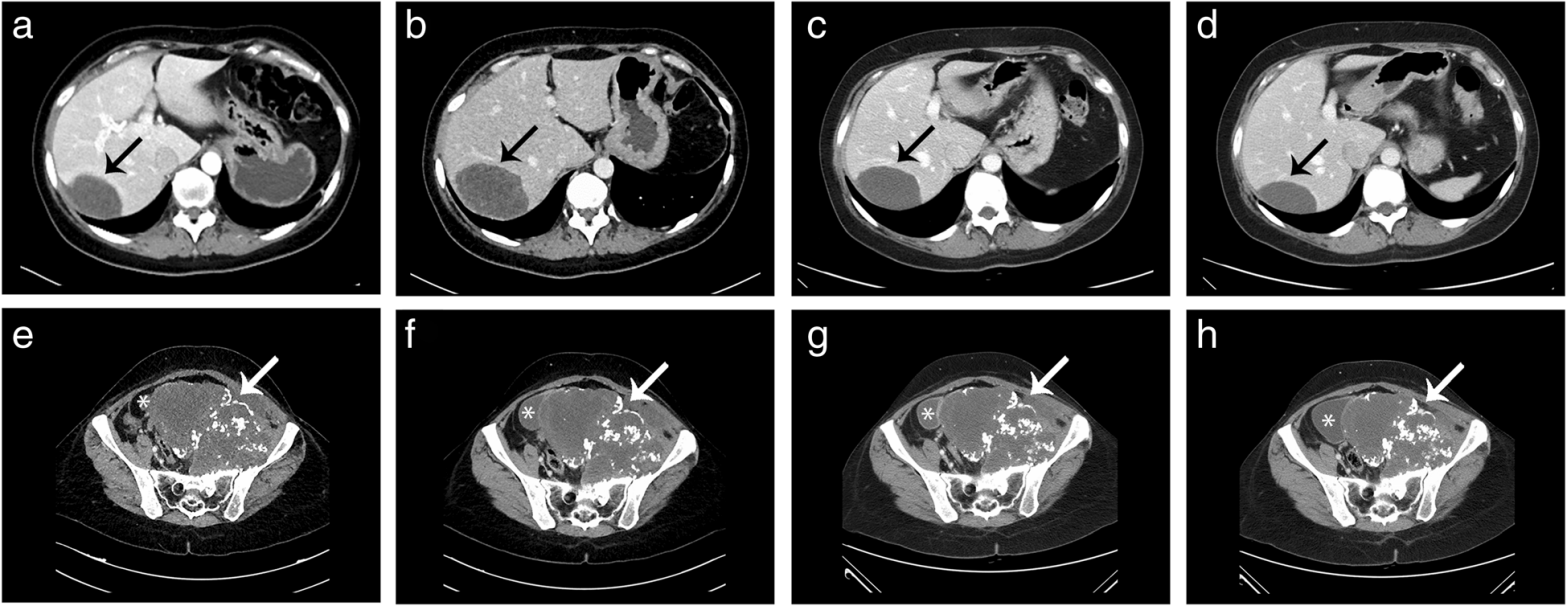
Contrast-enhanced CT scan of the liver and the pelvis (axial plane, abdomen window, venous phase). The progressive hepatic metastasis (black arrow) before (a) and after (b) chemotherapy with cisplatin/etoposide, then a RECIST response after 4 (c) and 9 (d) cycles of gemcitabine. Pelvis reports (e-h) appear stable (white arrow: primary tumour; asterisk: urinary bladder).
